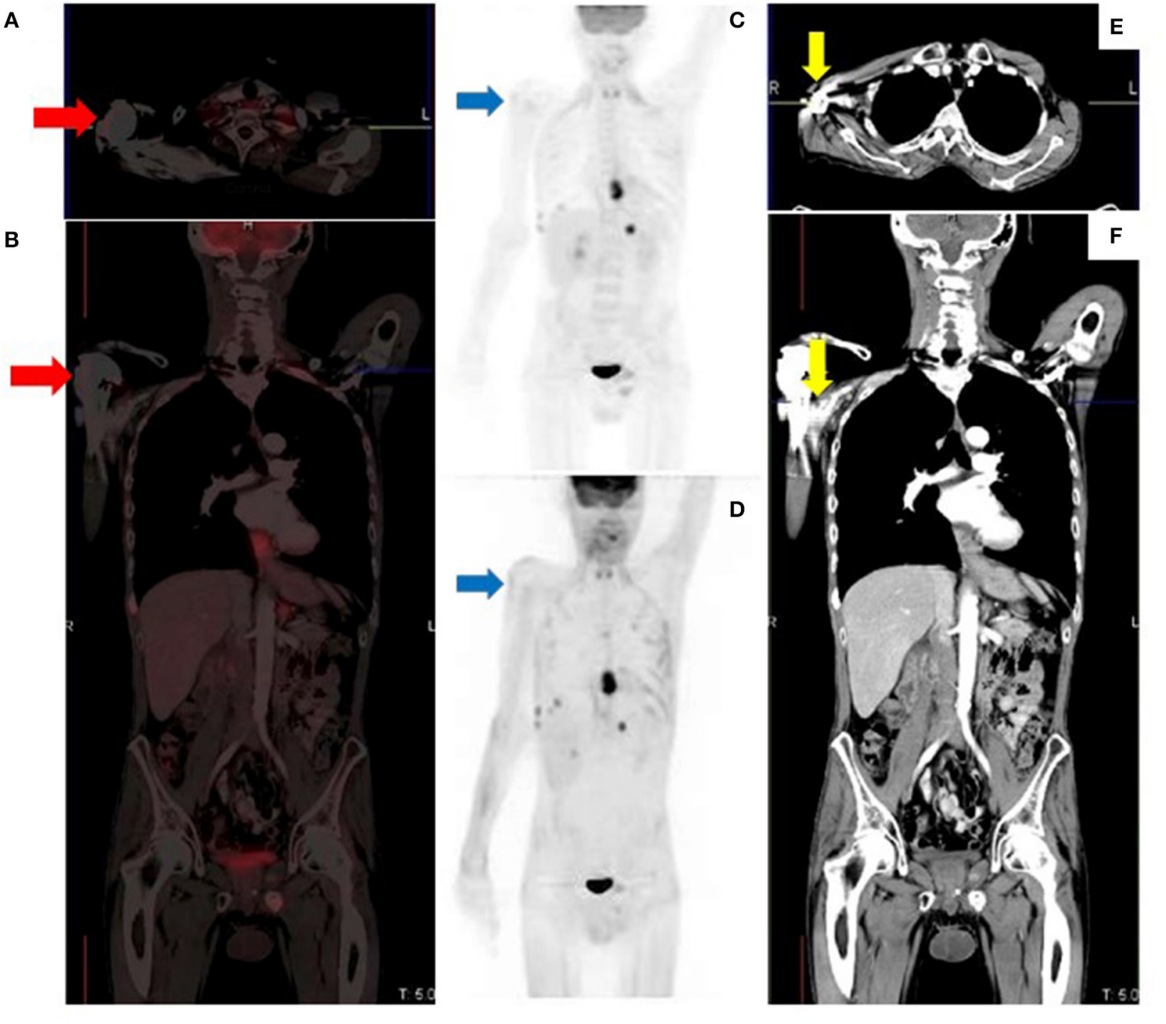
Metallic prosthetic right shoulder implant with overcorrection artifact resulting in mildly increased uptake (red arrows) on the fused (A,B) and attenuation corrected (C) images. There is no demonstrable peri-prosthetic uptake on the non-attenuation corrected image (D). The implant produces streak artifacts (yellow arrows) best appreciated on the CT images (E,F).
